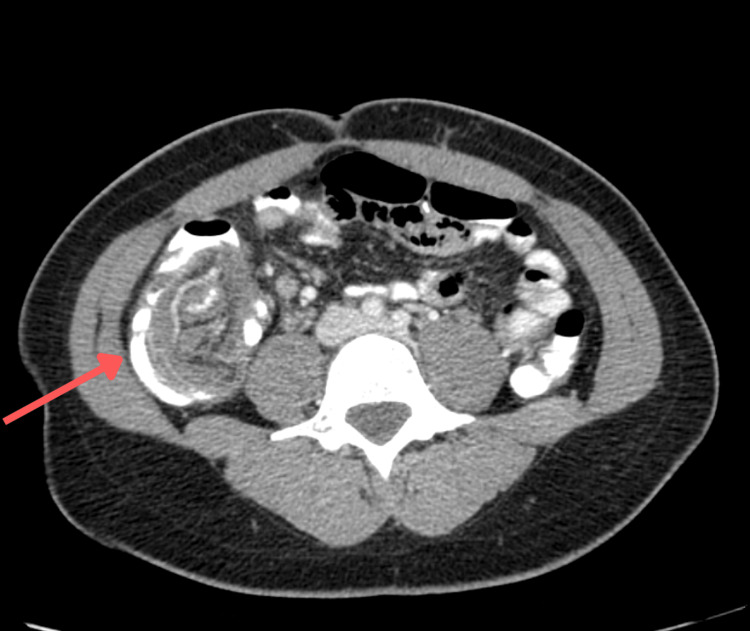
CT image of the abdomen and pelvis, cross-sectional view, with contrast. The red arrow points at an ileocolic intussusception. CT: computed tomography
